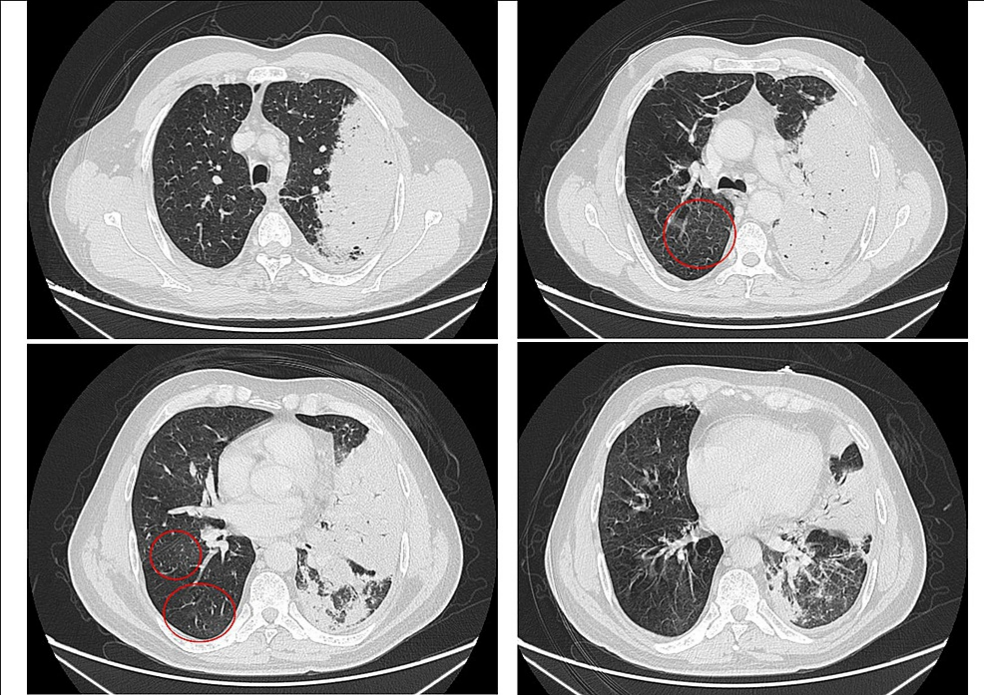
CT scan of the chest showing the opacity extending over the left lung with ground glass foci in the right lung during hospital admission
